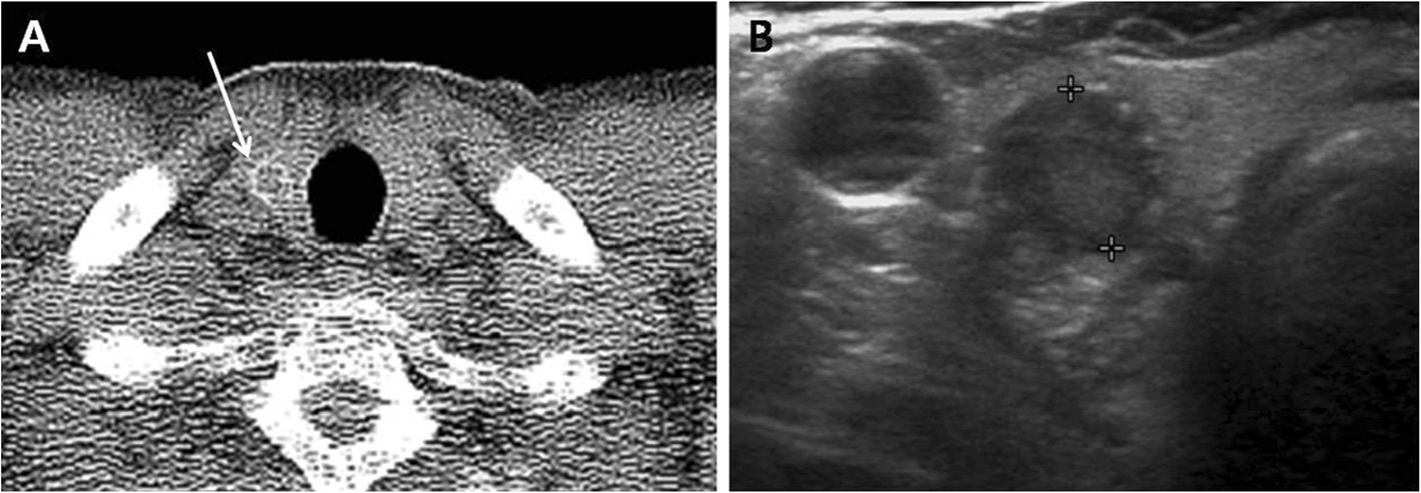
A 53-year-old man with papillary thyroid carcinoma. (A) Low-dose helical CT scan demonstrated a 9 mm hypodense nodule (arrow) with peripheral rim calcification in the right thyroid lobe. (B) Transverse US image of the right thyroid lobe showed a hypoechoicnodule (calipers) with peripheral rim calcification.
